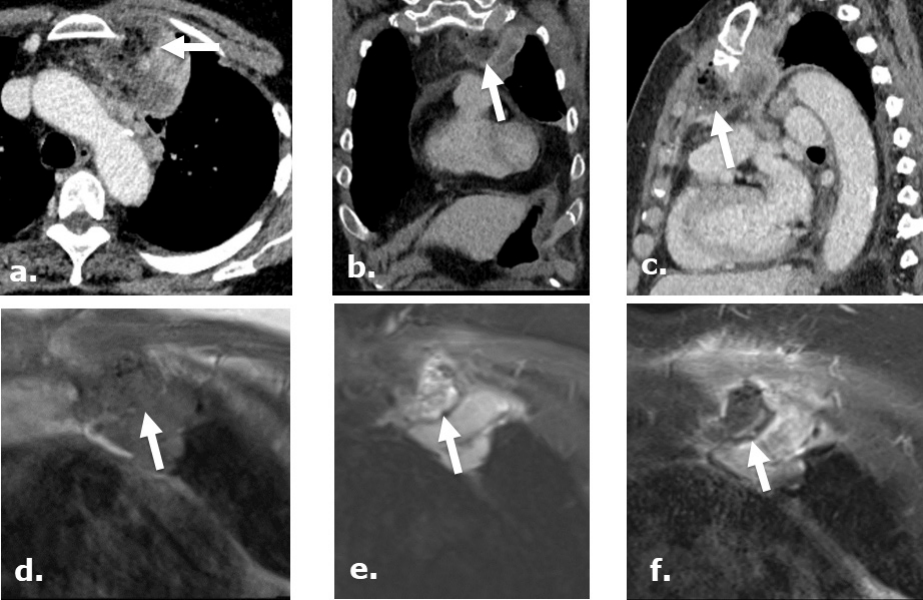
A 75-year-old female post-biopsy of a mediastinal mass. Contrast-enhanced CT images axial (a), coronal (b) and sagittal (c), day 7 post-biopsy, demonstrate heterogeneous, complex gas-containing collection medial to a biopsied mass, referring physician has confirmed the use of Surgicel during the procedure in this location. MRI images of the same region obtained status post biopsy day 12 demonstrate a collection isointense to muscle on coronal unenhanced T1 (d) and heterogeneous intensity focus on coronal STIR (e) with no enhancement in the retained Surgicel on coronal gadolinium enhanced T1-weighed (f). There are punctuate low intensity foci in the collection compatible with gas while surrounding chest wall and mass demonstrate enhancement.
